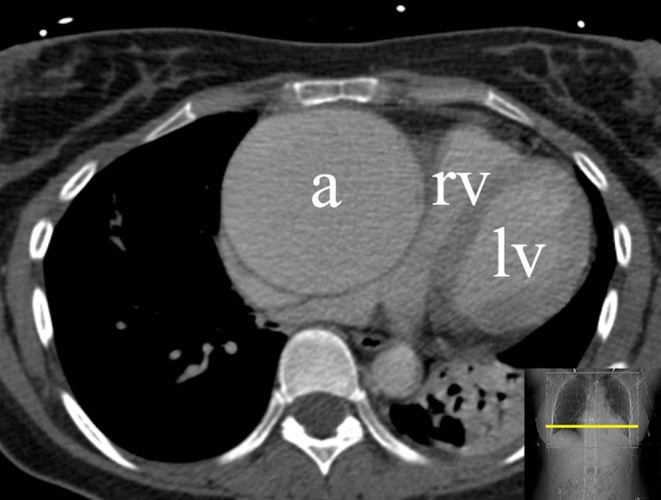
Thoracic CT showing a large intrathoracic mass. Note the equal densities of the aneurysm and the left ventricular lumen
